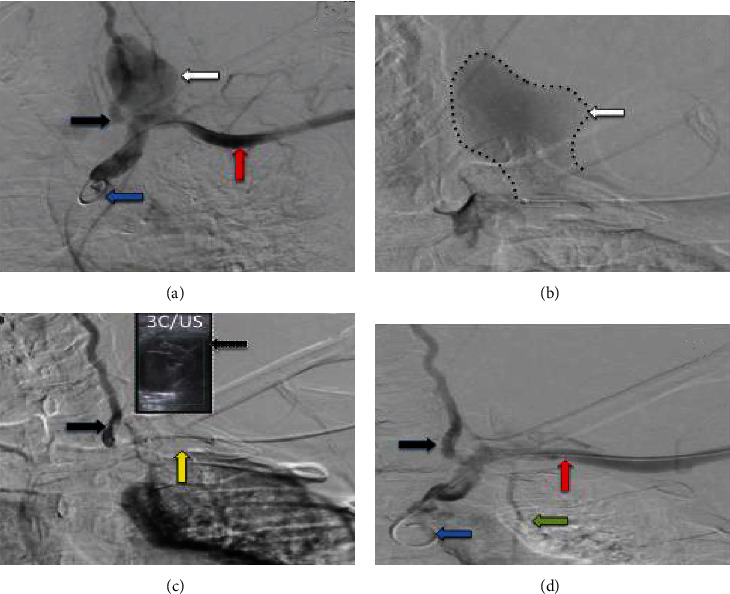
(a, b) Pigtail catheter (blue arrow) arteriogram showed pseudoaneurysm (white arrow) arising from the proximal part of the left subclavian artery (red arrow) distal to the origin of the left vertebral artery (black arrow). (c) Angioplasty balloon-tipped catheter (yellow arrow) covering the neck of the pseudoaneurysm. Bedside ultrasound documents the absence of the flow within the pseudoaneurysm by inflating the balloon, and injection of thrombin was done under ultrasound guidance ((c) and US). (d) Arteriogram after the thrombin injection shows no pseudoaneurysm, and it shows the left subclavian artery (red arrow), left vertebral artery (black arrow), and left internal mammary artery (green arrow).
